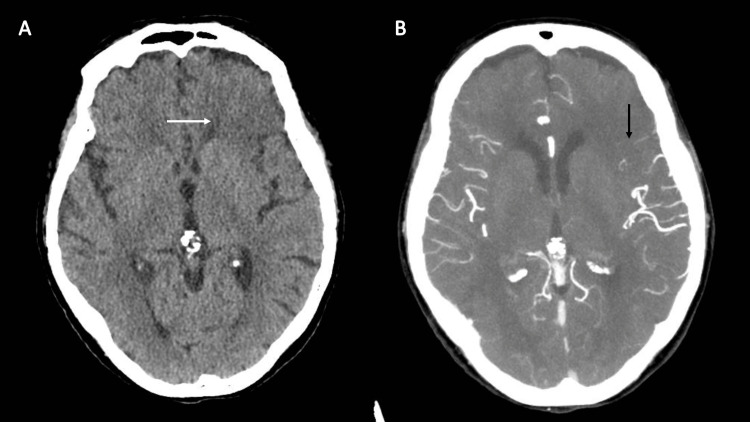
CT findings in Case 3 (A) Non-contrast head CT demonstrating subtle loss of gray-white differentiation in the left frontal cortex (white arrow); (B) CT angiography demonstrating abrupt cutoff in the superior left M2 distribution (black arrow).
